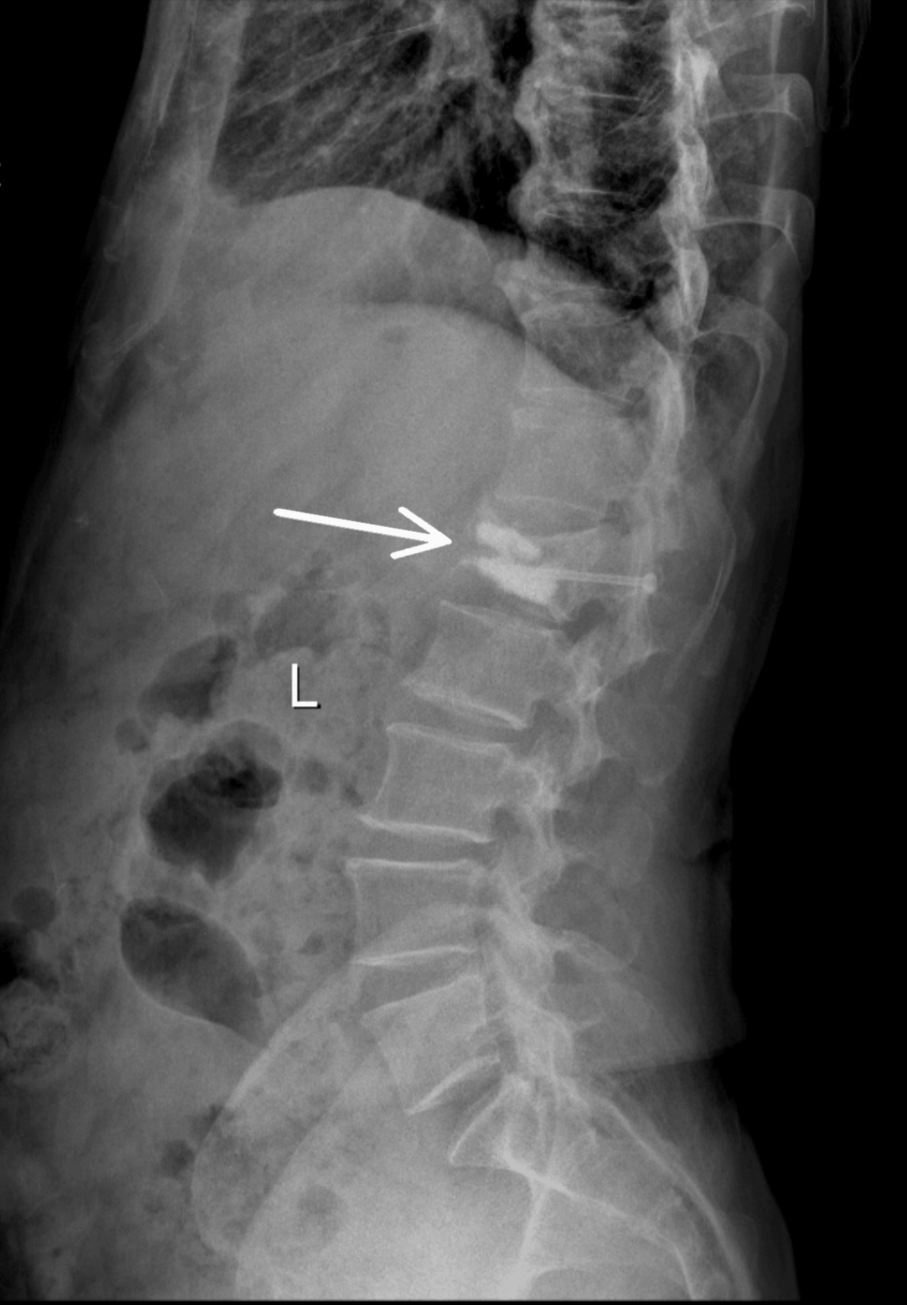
Lateral X-ray view of L1 vertebra after the surgical procedure.
